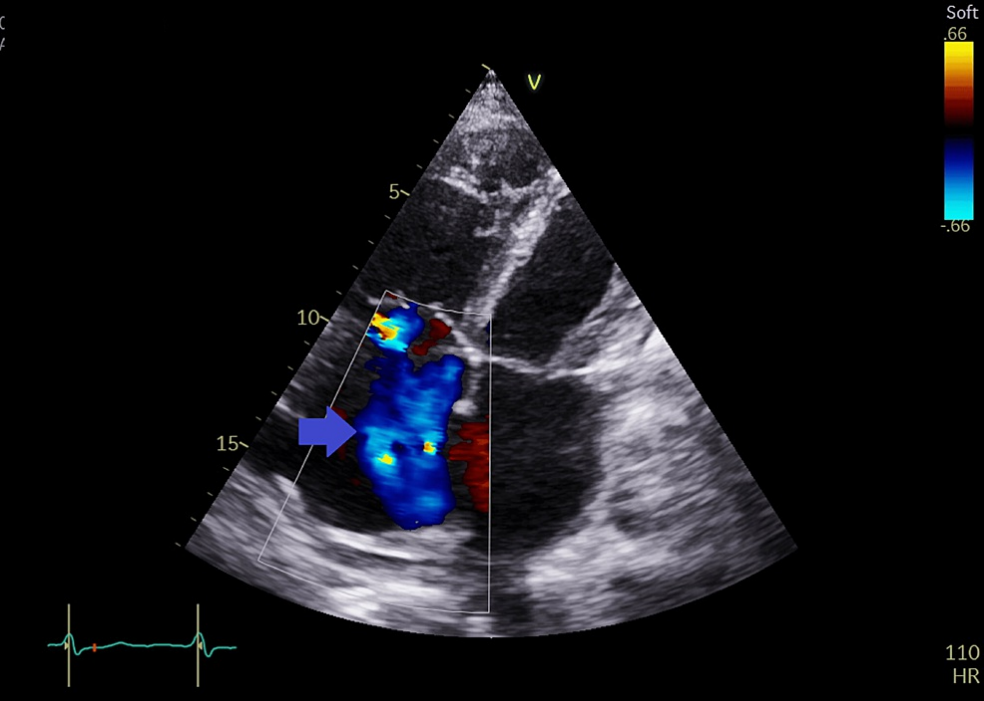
Apical four-chambers view with color coding of the tricuspid valve showing severe tricuspid regurgitation (blue arrow).
